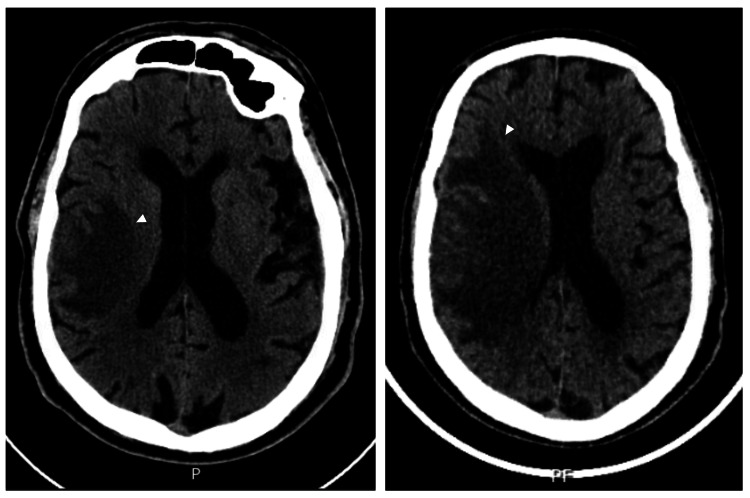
Cranioencephalic computed tomography (CT) findings during initial evaluation. Left: Non-contrast cranioencephalic CT at admission demonstrating a right frontoparietal hypodense lesion (white arrowhead) with associated mass effect, initially interpreted as acute ischemic stroke. Right: Repeat non-contrast cranioencephalic CT performed due to neurological deterioration, showing lesion progression (white arrowhead) with increased mass effect and surrounding vasogenic edema, raising suspicion of a space-occupying lesion.
